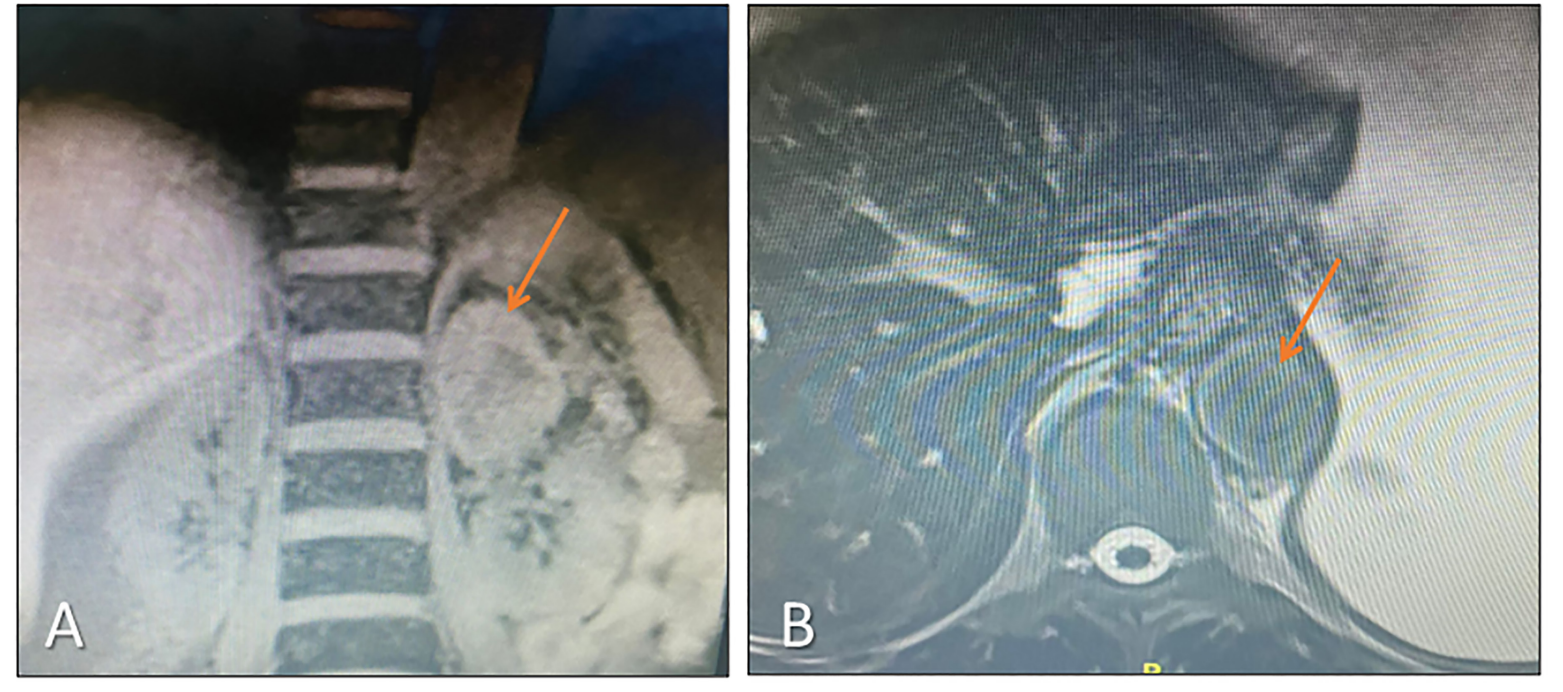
Left adrenal pheochromocytoma in a 63 yo patient. A: 6.3 cm lesion showing a cleavage plan with necrosis (MRI, coronal section). B: Chemical shift does not show loss of signal in the out-of-phase sequence (MRI, axial section).
